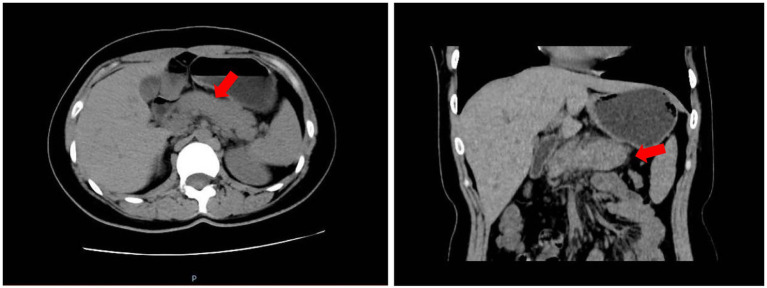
Abdominal CT (13 March 2024, 6 days after drug withdrawal) demonstrating near-complete resolution of peripancreatic exudates and normal pancreatic size.
